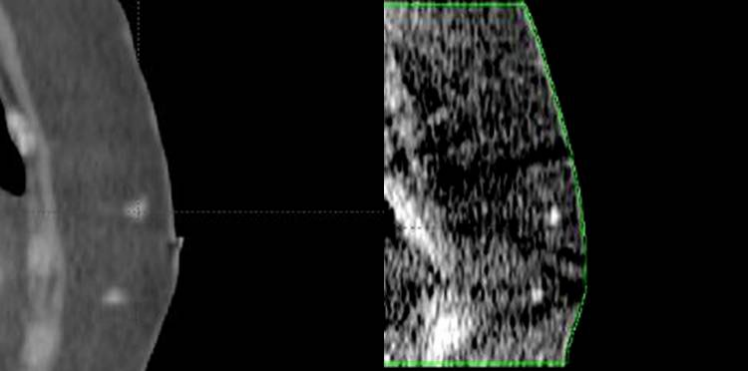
CT and kV CBCT images of two TraceIT markers in the breast. The CT image was acquired at 120 kVp using a GE LightSpeed with AEC; the CBCT image was acquired at 110 kVp, 259 mAs using a Varian OBI.
